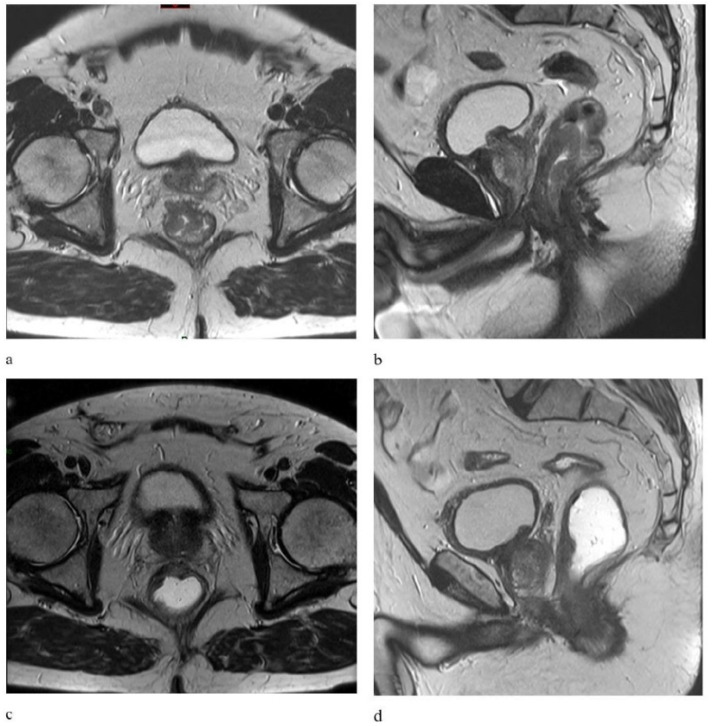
Axial and sagittal T2-weighted MR images show a distal rectal tumor with mesorectal fascia (MRF) infiltration in a patient with a clinical staged T3-N1 tumor before (a,b) and after chemoradiotherapy (c,d) showing a locally advanced rectal cancer with a good response to neoadjuvant chemoradiotherapy. MR, magnetic resonance.
